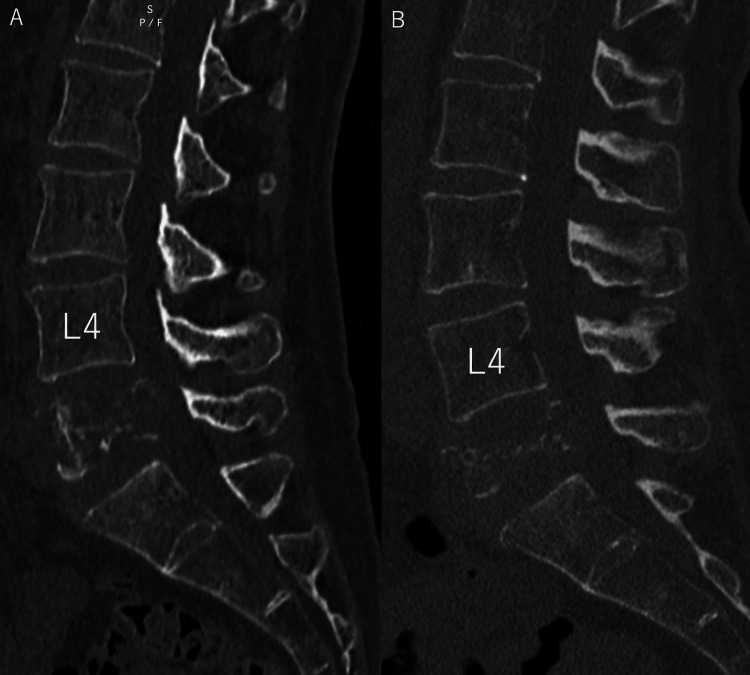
(A) Sagittal CT image at initial examination. (B) Sagittal CT image one month after the initial examination. A. The L5 vertebral body showed osteolytic changes and was considered a metastatic spinal tumor. B. The L5 vertebral body showed progressive destruction and osteolytic changes.
